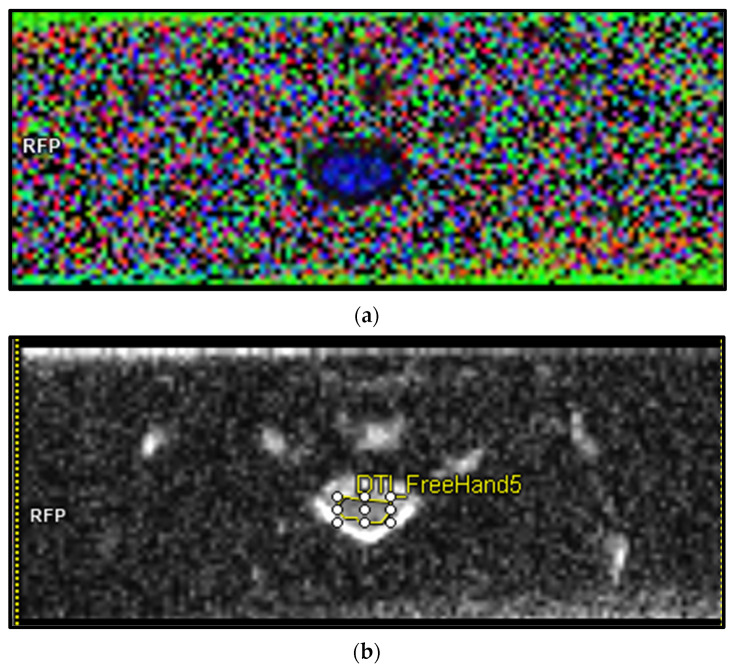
Preliminary data obtained from a healthy recruit using 3T MAGNETOM PRISMA MRI scanner with rFOV DTI ZOOMit sequence. From our standardisation work we have found rFOV to offer better visibility, better signal-to-noise, and less susceptibility and motion artifacts when compared to full field of view (fFOV) sequences. (a) axial Col-FA map of C4/5 cervical spinal-cord (red = left-right, blue = supra-inferior green = antero-posterior). (b) axial ADC map of C4/5 cervical spinal cord. Abbreviations: ADC, apparent diffusion coefficient; DTI, diffusion tensor imaging; Col-FA, colour fractional anisotropy; MRI, magnetic resonance imaging; rFOV, reduced field of view; ROI, region of interest.
